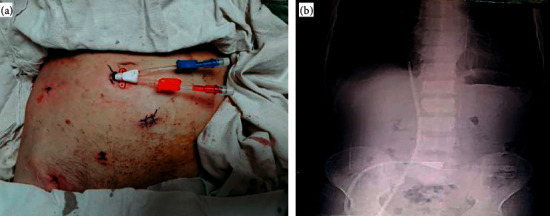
Right iliac approach of the tunneled cuffed catheter. (a) shows the position of catheter exit site below the umbilicus away from the groin making a low possibility of exit site infection. (b) Plain X-ray of the chest and abdomen shows the smooth curve of the tunnel, and the catheter tip is directed upward in the inferior vena cava till the cavo-atrial angle.
